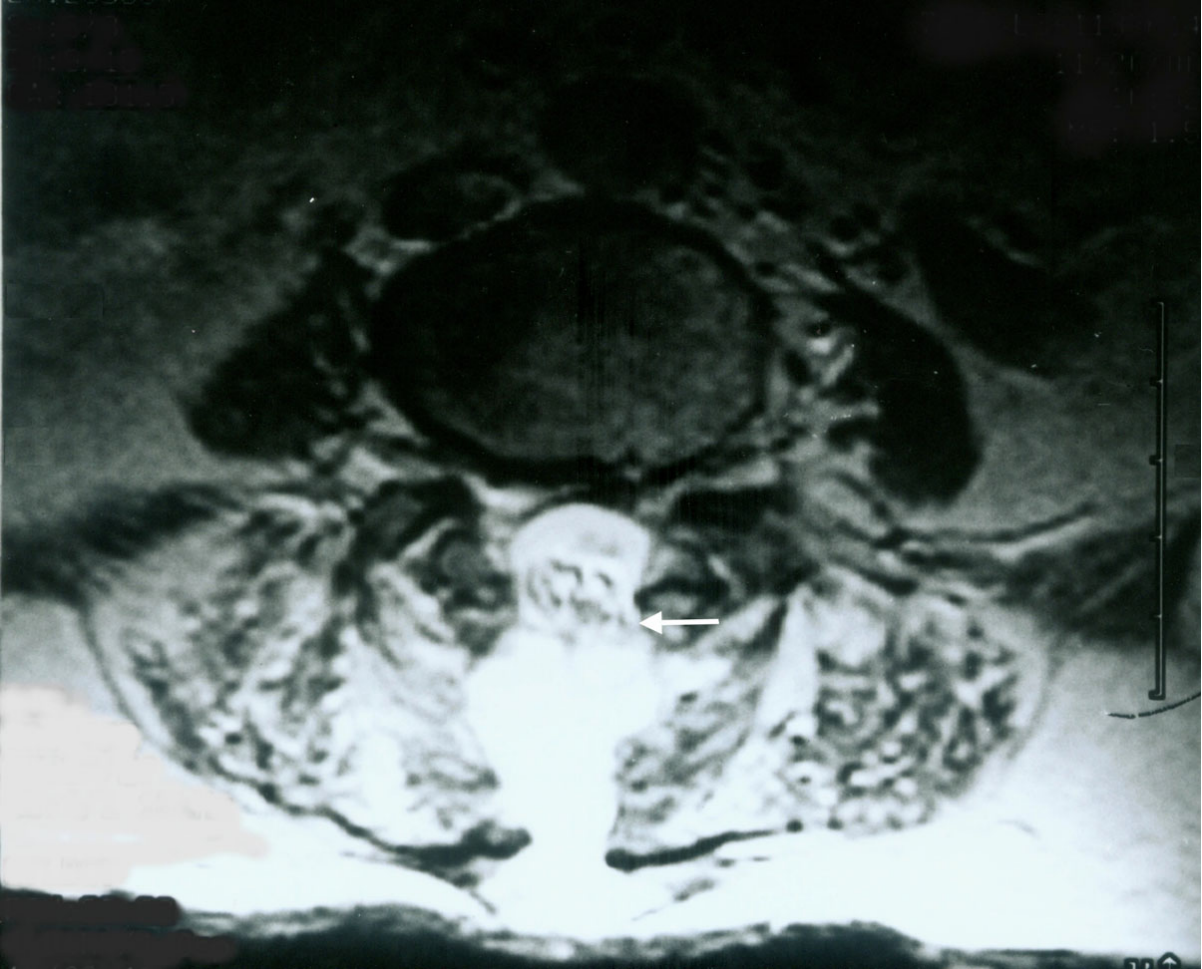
Nerve roots herniating into a pseudomeningocele. A 78-year-old male underwent laminectomy and discectomy for L4--L5 disk herniation. A giant pseudomeningocele (length, 9 cm; width, 5 cm; depth, 3 cm) developed after surgery. Nerve roots herniating through the dural and arachnoid tears and into the pseudocyst was observed on axial MRI (arrow).
